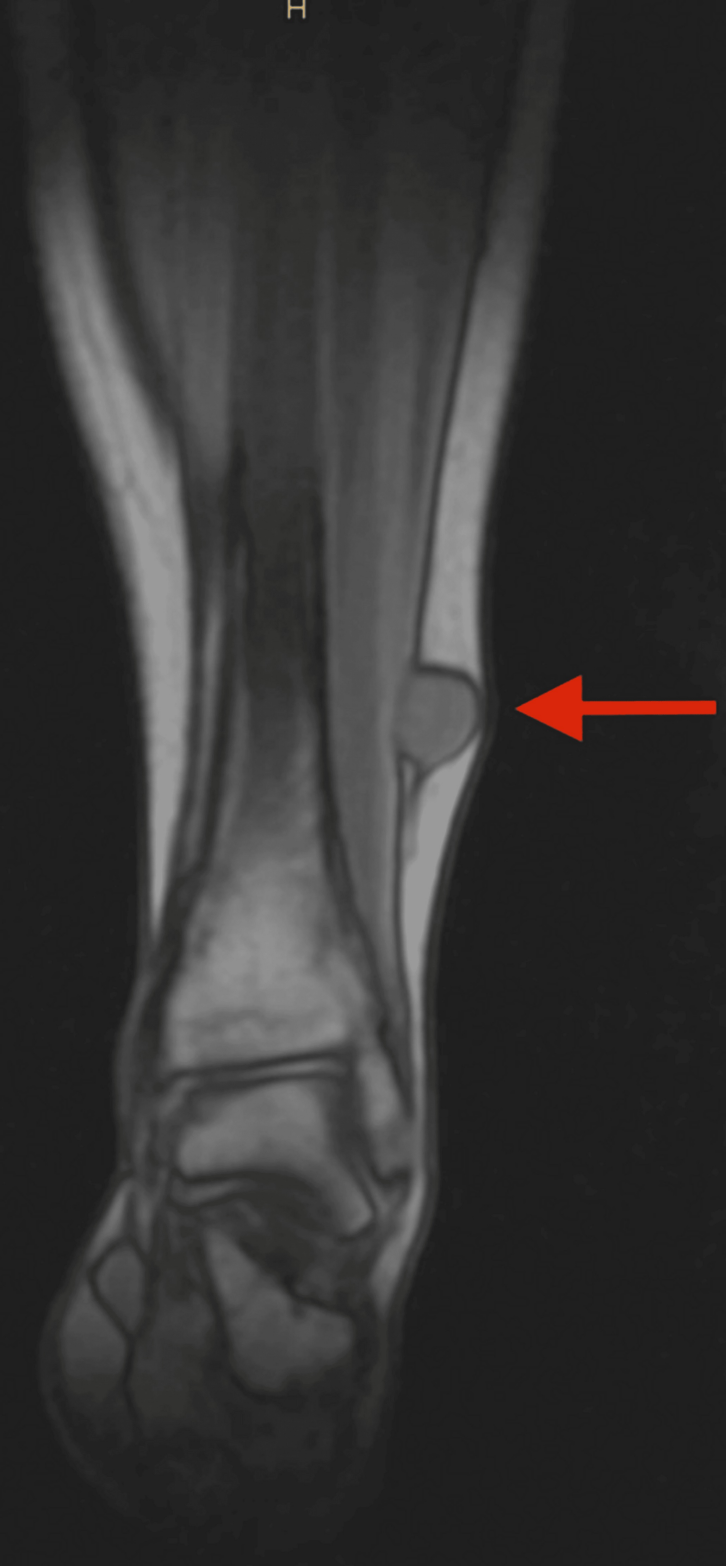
Pre-operative MRI of the left tibia showing a well-defined, oval-shaped mass, measuring 16x22mm (arrow).
